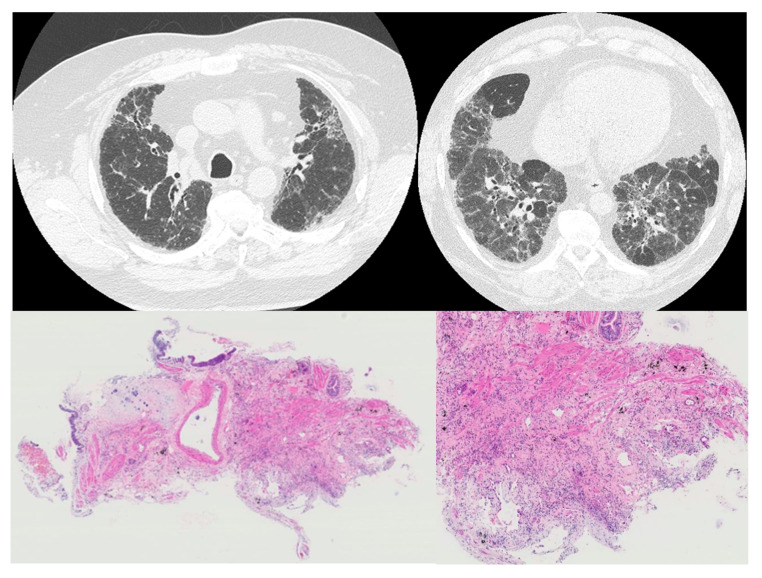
An fHP case in which airway disease was observed radiologically but not pathologically. High-resolution computed tomography (HRCT, (upper)) showed centrilobular nodules in the upper lobe and air trappings in the lower lobe. In transbronchial lung cryobiopsy (TBLC) pathology (lower), dense fibrosis with architectural destruction was observed in the peripheral of lobules; however, airway disease was not observed in TBLC.
